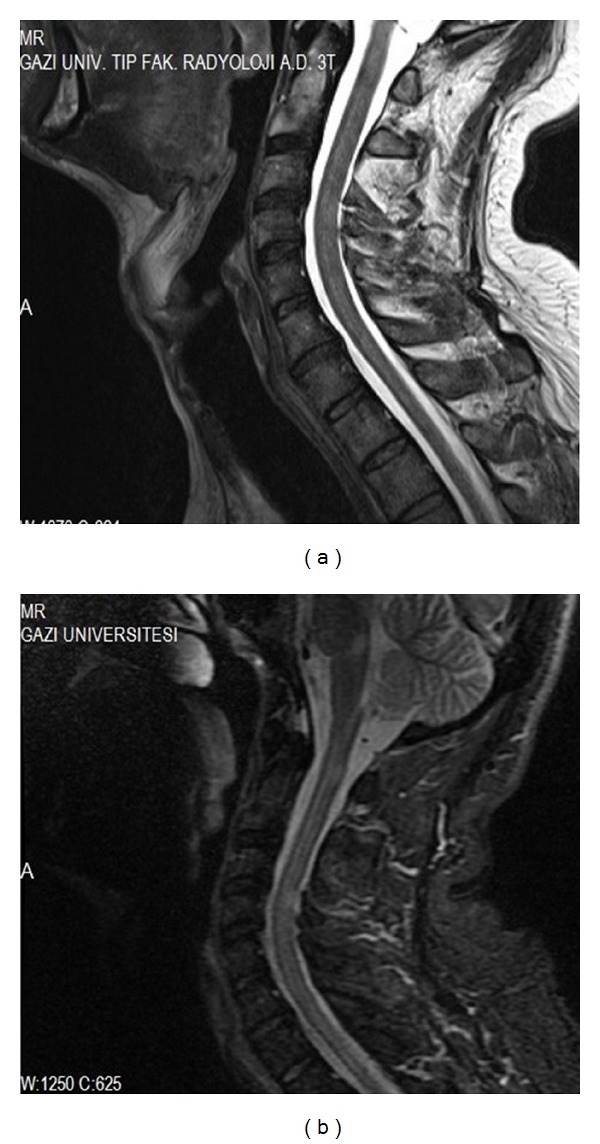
(a) Normal cervical MRI, T2 weighted sagittal section, (b) demyelinating posterior and posterolateral cord lesions in C1, C4, and C5-6 intervertebral disc levels and in upper thoracic segments, T2 weighted sagittal section, and control cervical MRI.
